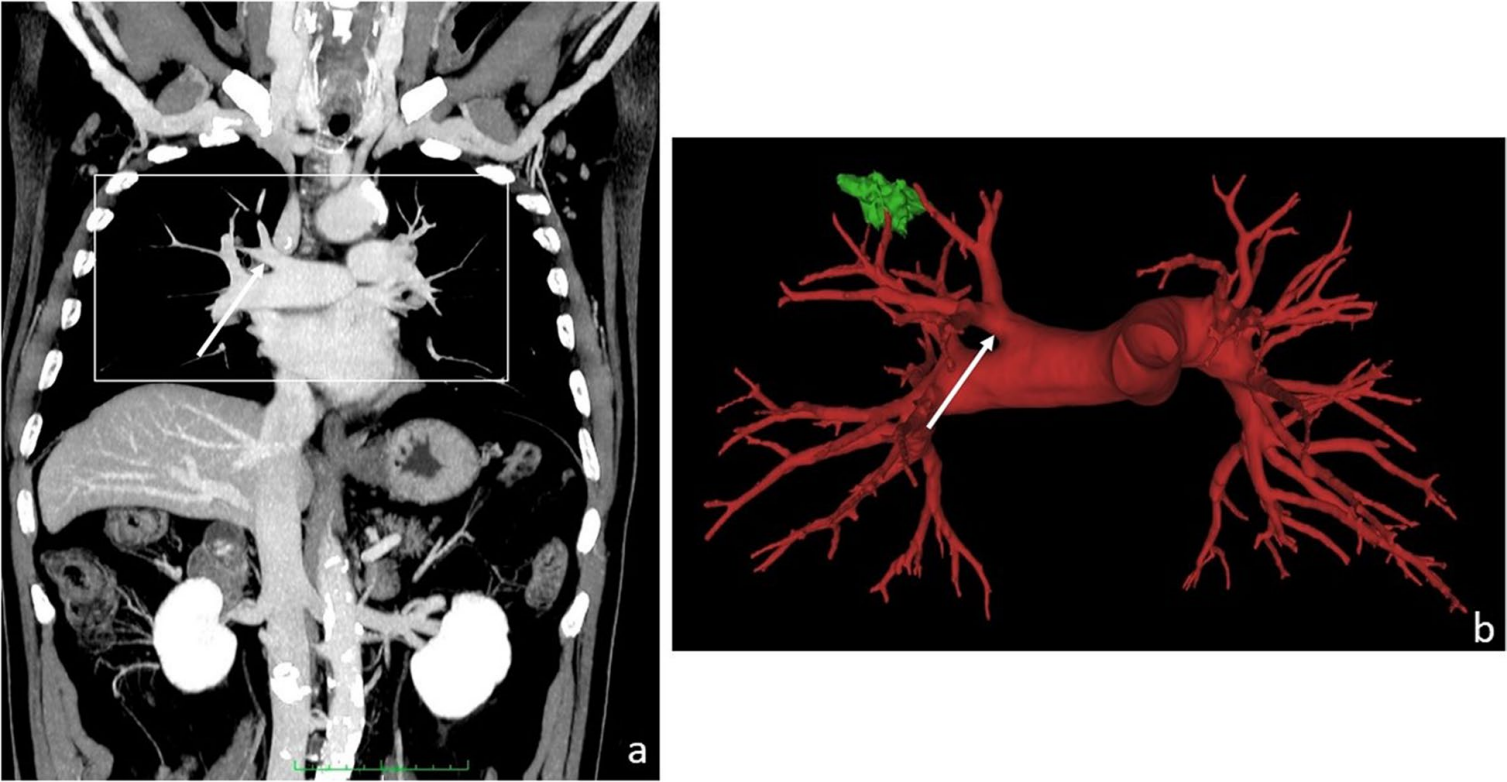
Coronal maximum intensity projection reconstruction of the contrast-enhanced computed tomography scan (a), containing a white box indicating where the pulmonary vessels were specifically evaluated, and HG of the pulmonary vascular anatomy (b) in a patient candidate to right upper lobe lobectomy for lung cancer (green lesion). The radiologist and surgeon evaluation where concordant with the reference standard (surgery) in indicating a single artery for the upper lobe (white arrow in a and b)
